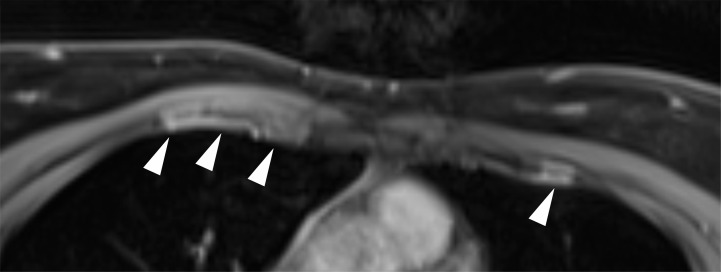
MRI demonstrated perichondritis at the level of the third and fourth ribs. Axial fat-saturated T1-weighted images after administration of intravenous contrast agent (gadolinium): Linear contrast enhancement of the costal cartilage on both sides at the level of the third and fourth ribs (white arrowheads), predominantly at the costochondral junction, consistent with perichondritis. Note also the subtle diffuse enhancement of the adjacent soft tissue on the right side.
